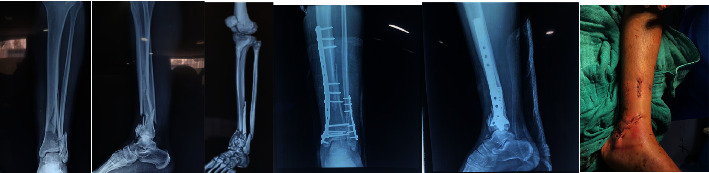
Pre- and postoperative radiographic and clinic check-up of a 42-year-old patient operated on for a 43-C3 fracture using a minimally invasive approach.
